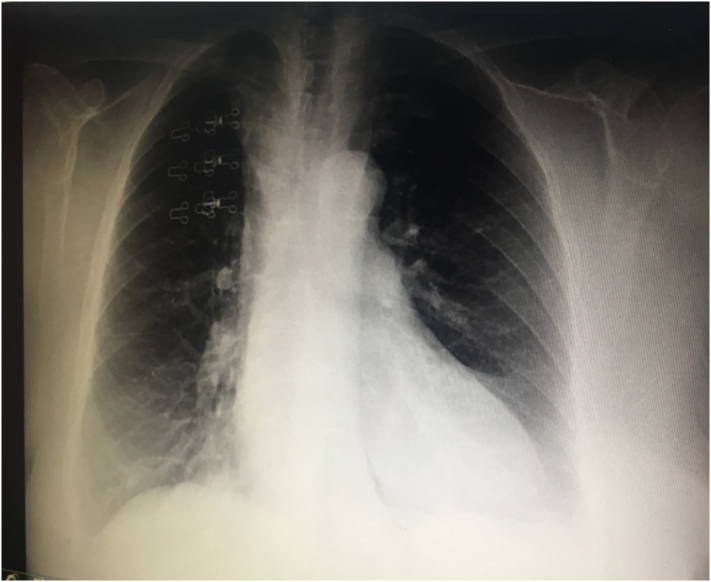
Post-operative chest x-ray with a centrally located trachea and no mediastinal mass.
